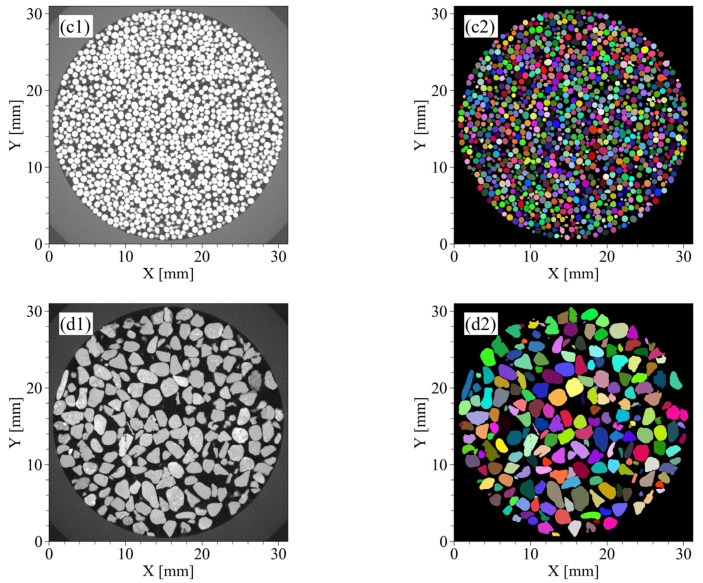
CT images and segmented images of four materials poured into acrylic glass columns: (a1,a2) RP1 and (b1,b2) RP2 (resin particles made by a 3D printer), (c1,c2) GBs (glass beads), and (d1,d2) KS (Kashima–Keisa natural sand). The left columns are CT images, and the right columns are segmented images.
